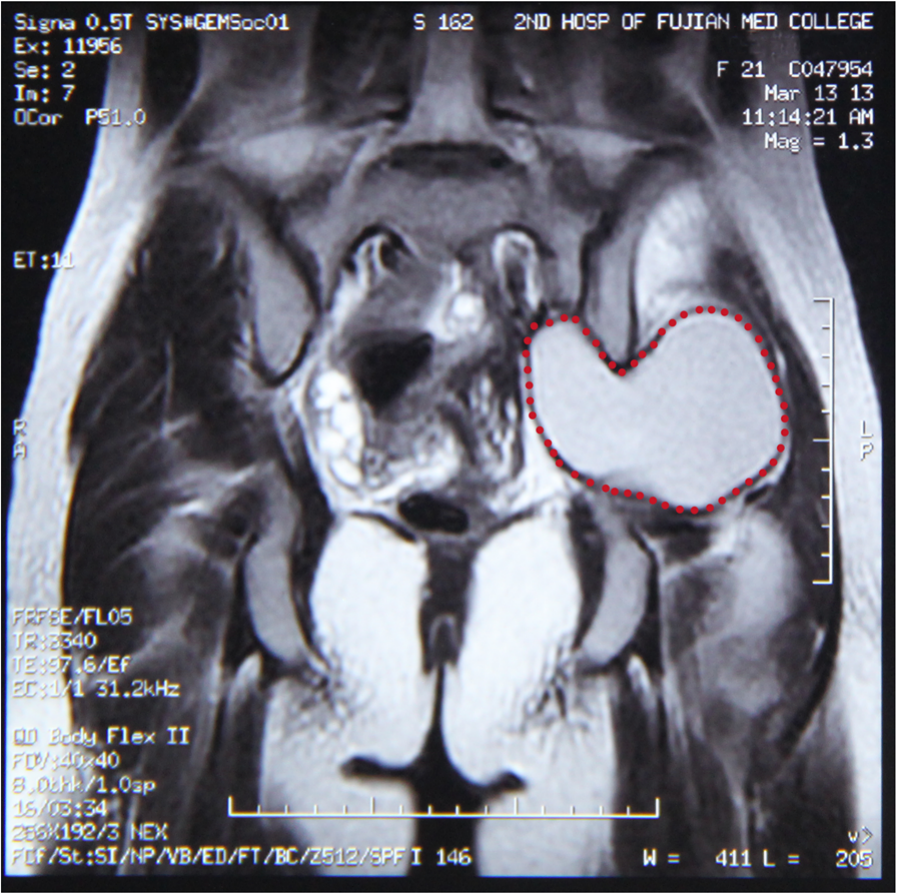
T2-weighted MRI image of the lesion. A cyst full of fluid was observed in the pelvis and extended to the left buttock through the greater sciatic foramen (pink dotted circle; heart-shaped area)
